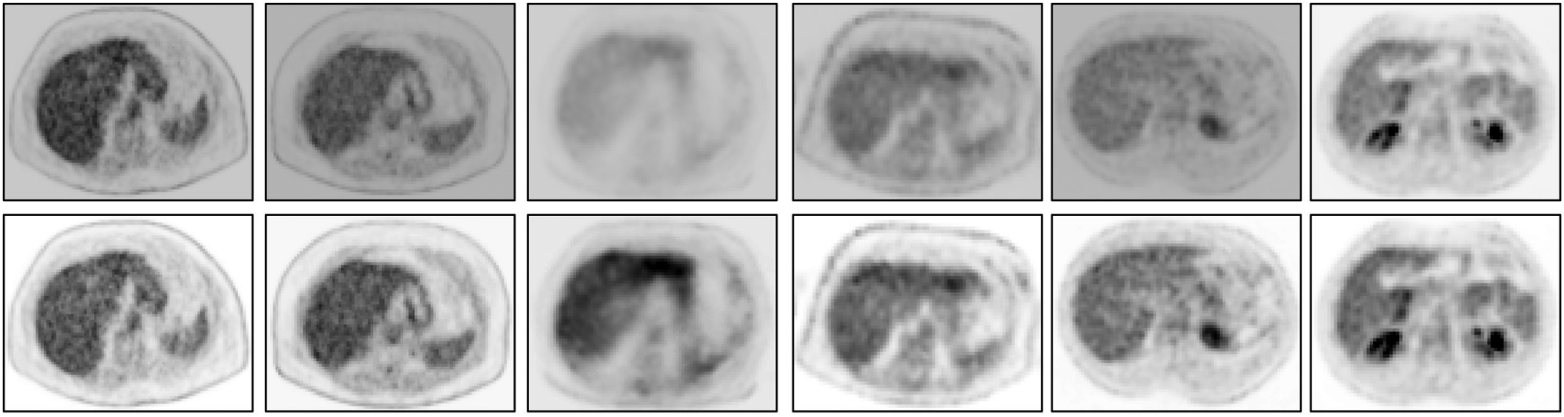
An abdominal SUV PET image slice selected from each of 6 3D body torso images *I* in $I_{n}$ before standardization (top row) and after standardization (bottom row). In each row, the images are displayed at a fixed gray map adjusted to the first image in the row.
